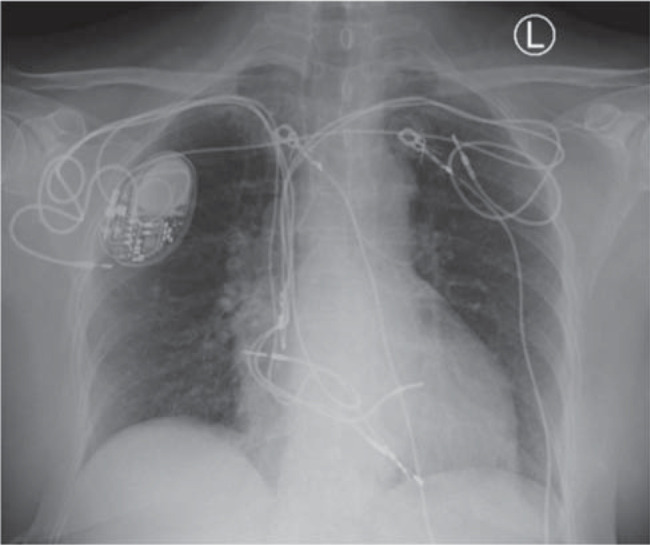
Chest X-ray showing right-sided pacemaker with 3 right-sided leads and 2 left-sided leads, including 1 tunneled across the chest over to the device on the right; also, note that a total of 5 leads pass through the superior vena cava.
